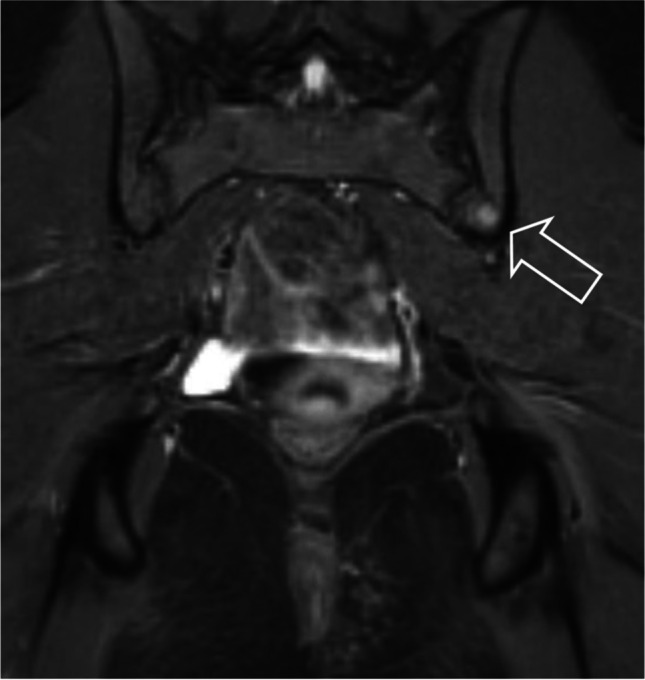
Follow-up coronal T2 magnetic resonance image of the pelvis in a 14-year-old girl (patient 2), 17 months after completion of chemotherapy, shows a small area of high signal intensity (*arrow*)
